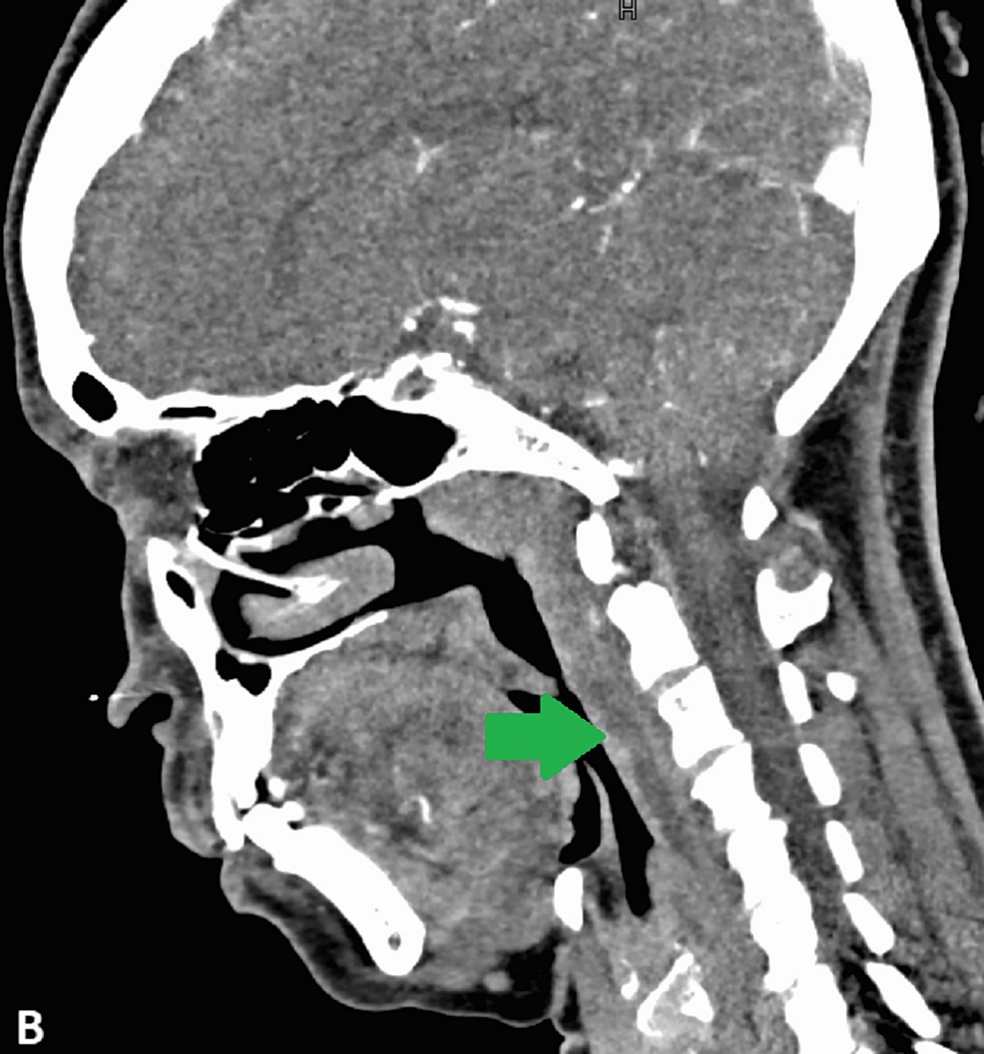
Contrast-enhanced computed tomography (CT) of the neck (sagittal view) Prevertebral effusion can be seen extending down up to the C4 vertebral body (green arrow).
